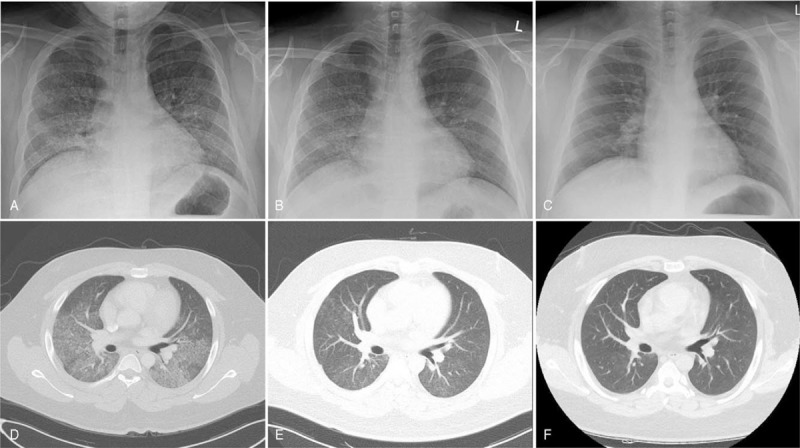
(A, D) On admission, chest radiographs showed diffuse opacities in both lung fields, and chest CT scans showed multiple small nodular and ground-glass opacities in both lung fields. (B, D) Ten days after completion of methylprednisolone pulse therapy, interval improvement of pulmonary lesions was seen. (C, E) Two months after hospital discharge, chest radiograph and CT scans showed complete resolution of previous lesions. CT = computed tomography.
